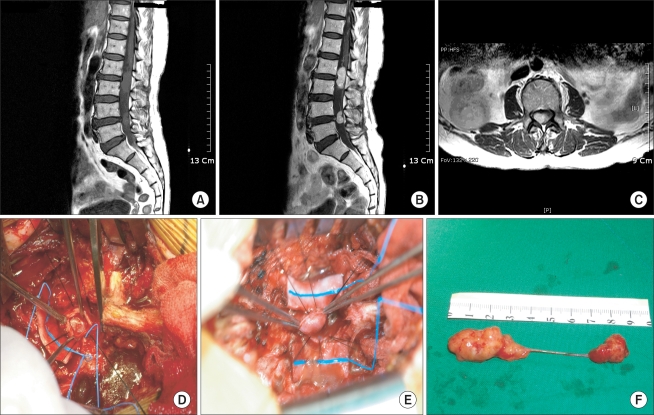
Seventy-four-year-old female with intradural extramedullary schwannoma with back pain and a sensory decrease. (A) T1-weighted sagittal MR image shows two homogenous iso-signal intensity masses. The tumor is located at the L2-3, 4-5 level with dumbbell shape for-mation. (B) Gadolinium enhanced T1-weighted sagittal MR image shows marginal enhancement of heterogeneous high signal intensity mass. (C) Gadolinium enhanced T1-weighted axial MR image shows space occupying intradural extramedullary mass compressing spinal cord to the left side. (D, E) Intra-operative findings of intradural extramedullary mass excision. (F) Extracted tumor showing a well encapsulated masses measuring 3.5 × 1.5 × 1 cm and 1.5 × 1 × 1 cm connected with linear stock.
